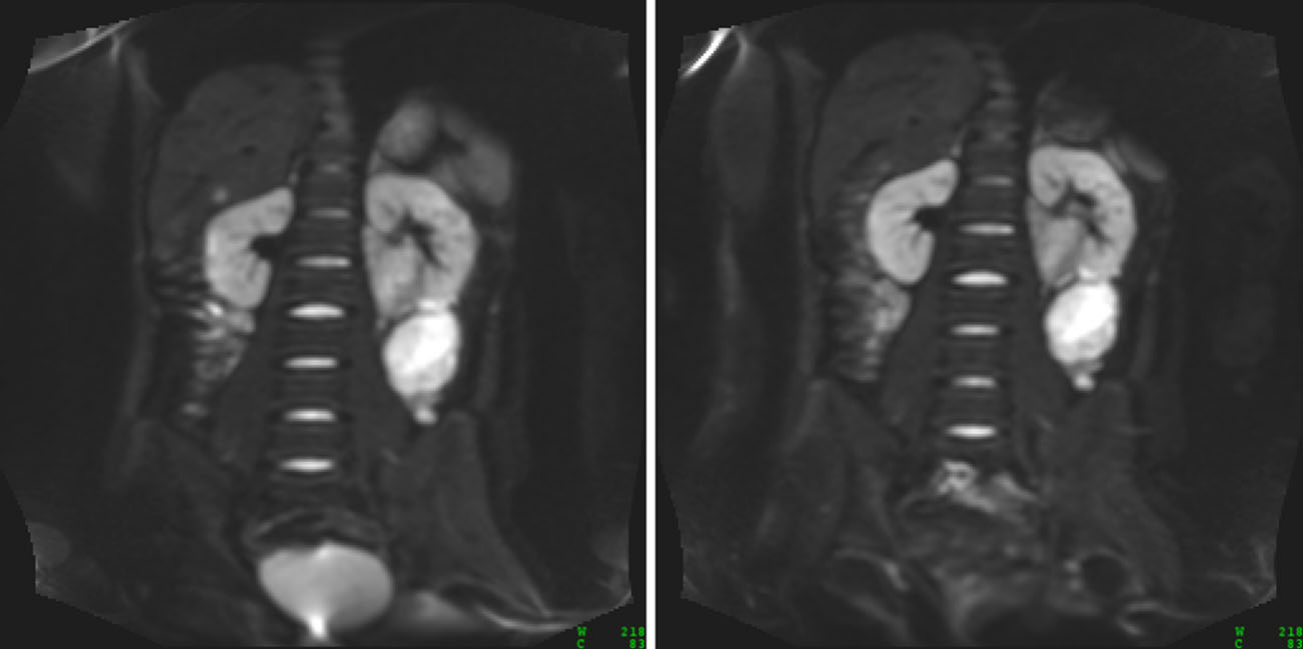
Representative images (*b* = 100 mm^−2^ s) from repeated DWI scans (*left* day 0, *right* day 1) of a patient with rhabdomyosarcoma, showing good visual agreement of patient/tumour positioning
